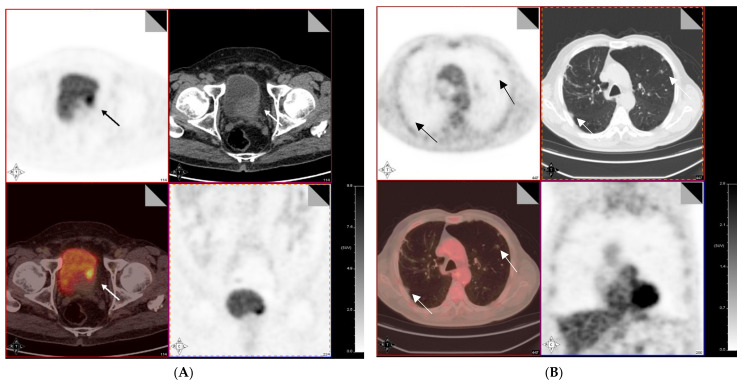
Transaxial FDG-PET with hydration (upper left), CT (upper right), fused (lower left), and MIP (lower right) of a patient with MIBC during staging before treatment. Focal intense FDG uptake on the left postero-lateral wall (solid arrow), without any other pathological uptake out of the bladder (A). In particular, small bilateral lung nodules did not show significant metabolism (B). The patient was submitted to cystectomy with wide lymphectomy, demonstrating pT4N0 MIBC. Lung nodules still persist as stable in the following TC.
